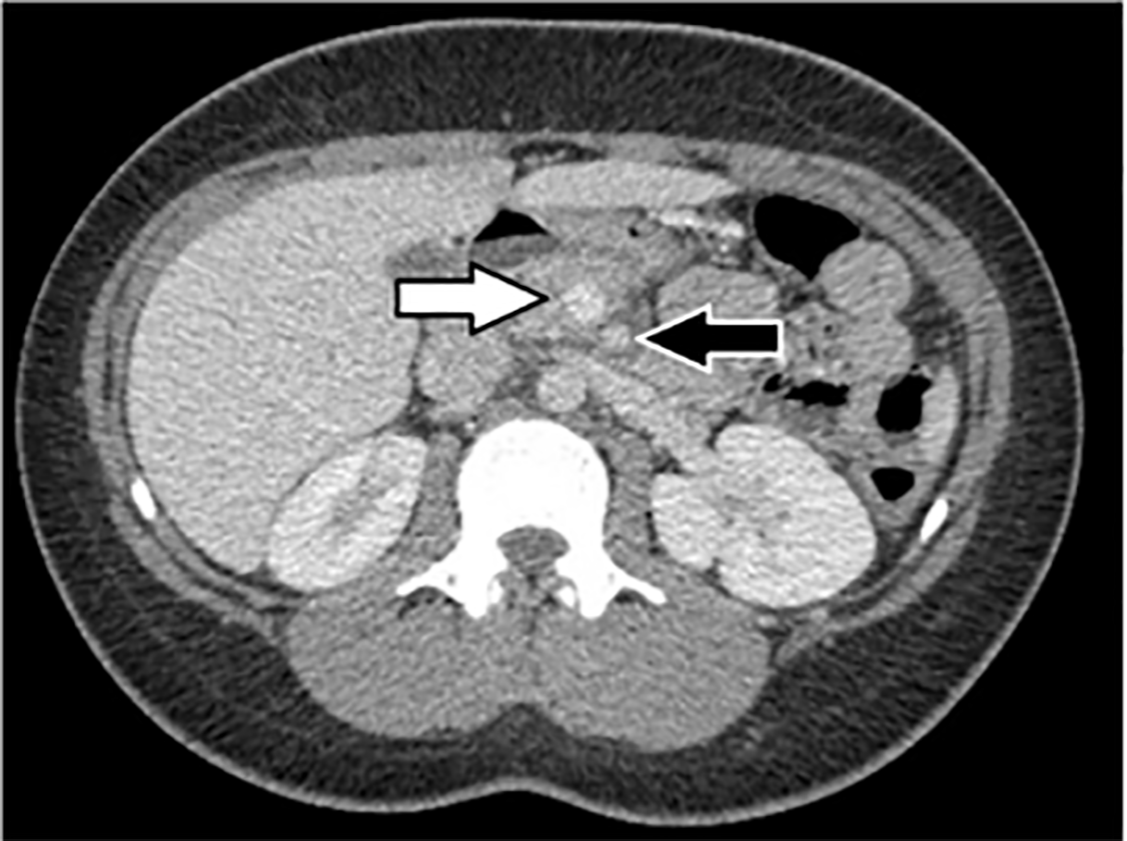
This axial computed tomography image shows the normal appearance of the superior mesenteric artery (black arrow) and just adjacent to it, the superior mesenteric vein (white arrow).
